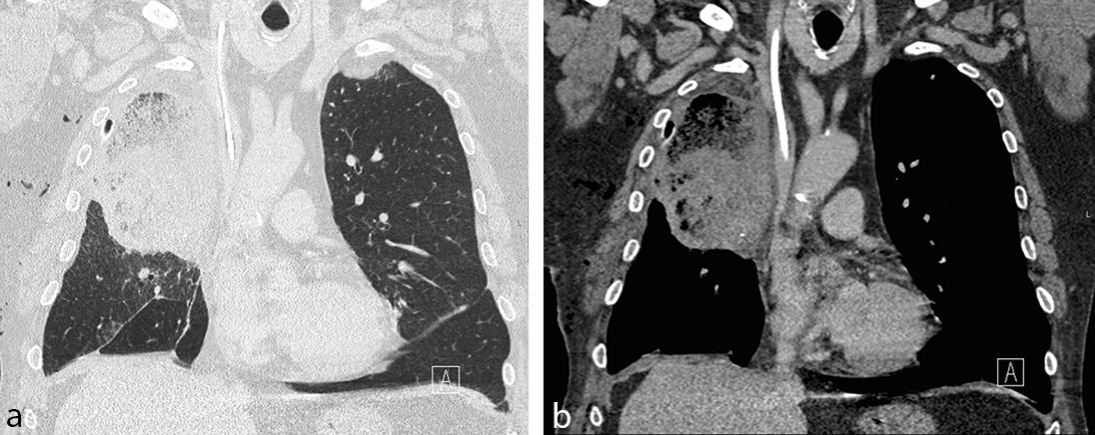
Coronal reformat of the post-operative contrast-enhanced CT scan. (a) Lung window setting. The middle lobe is expanded and superiorly located with extensive ground-glass consolidation. (b) Soft tissue window setting. The middle lobe is expanded and superiorly located with extensive ground-glass consolidation.
